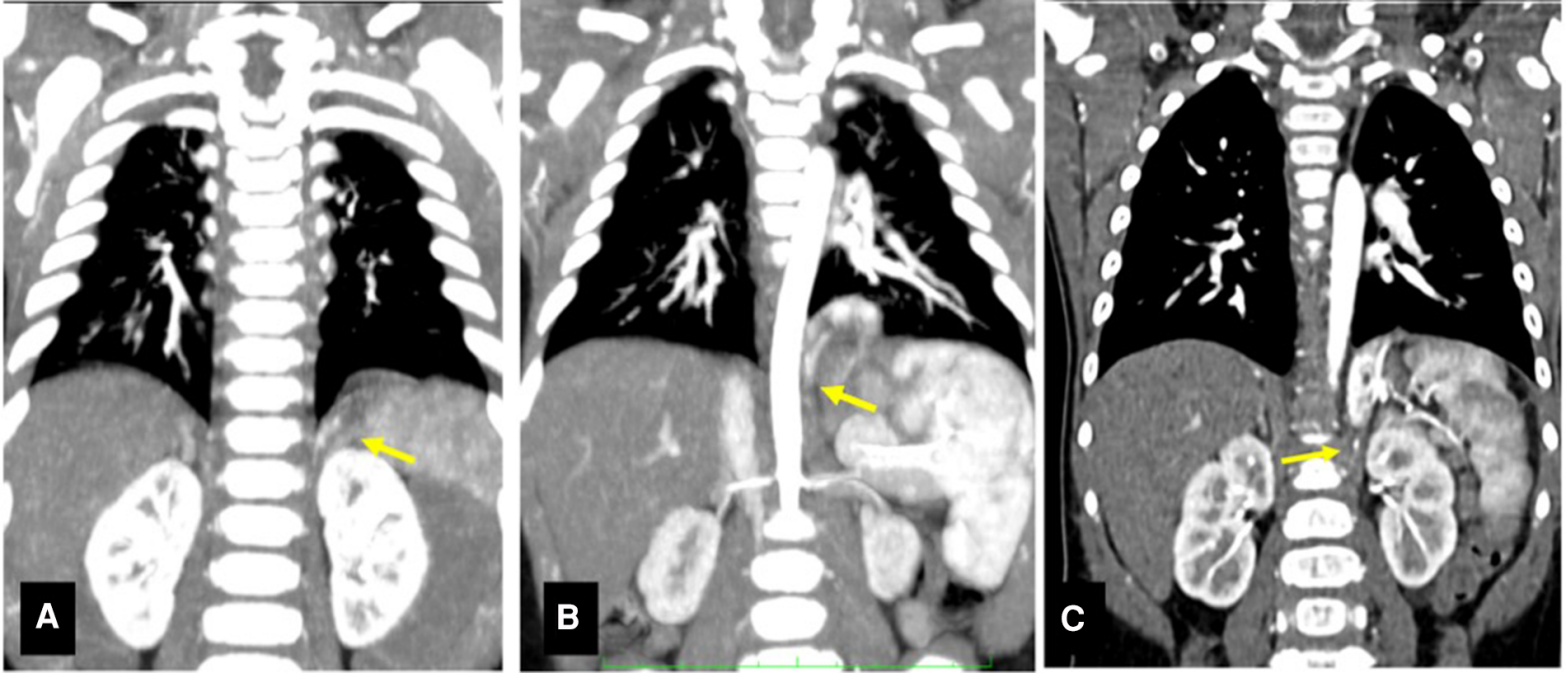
CT scans of presented cases. (A) CT scan Case 1. Structural alteration in the left lower lobe with increased parenchyma opacity, no signs of ventilation (1.5 cm × 2.7 cm × 1.5 cm), extending within the diaphragm. Arrow: small diaphragmatic arterial vessel originating from the celiac trunk is visible. (B) CT scan Case 2. Solid formation in the left costo-vertebral diaphragmatic angle (2.0 cm × 2.0 cm × 1.7 cm), regular margins and no clear connection with the bronchial tree. Arrow: artery originating from the celiac trunk (4.5 mm below its aortic origin). (C) CT scan Case 3. Solid intradiafragmatic mass with regular margins (3.3 cm × 1.5 cm × 2.9 cm). Arrow: systemic arterial supply with arteries from the celiac trunk.
